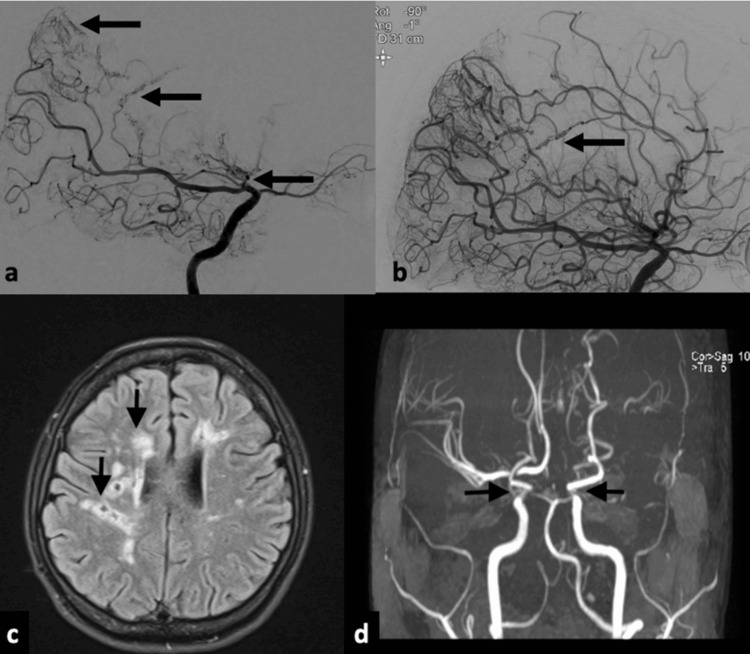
(a,b) Digital subtraction angiography of the patient demonstrates occlusion of the right ICA and collateral flow from posterior cerebral artery branches to the middle and anterior cerebral arteries (black arrows). (c) Brain MRI before the rituximab treatment showed multiple, small, chronic, ischemic foci of white matter (black arrows). (d) Brain TOF MRI angiography revealed severe stenosis of the right ICA and total occlusion of left ICA at the supraclinoid segment (black arrows) MRI: magnetic resonance imaging; TOF: time-of-flight; ICA: internal carotid artery
